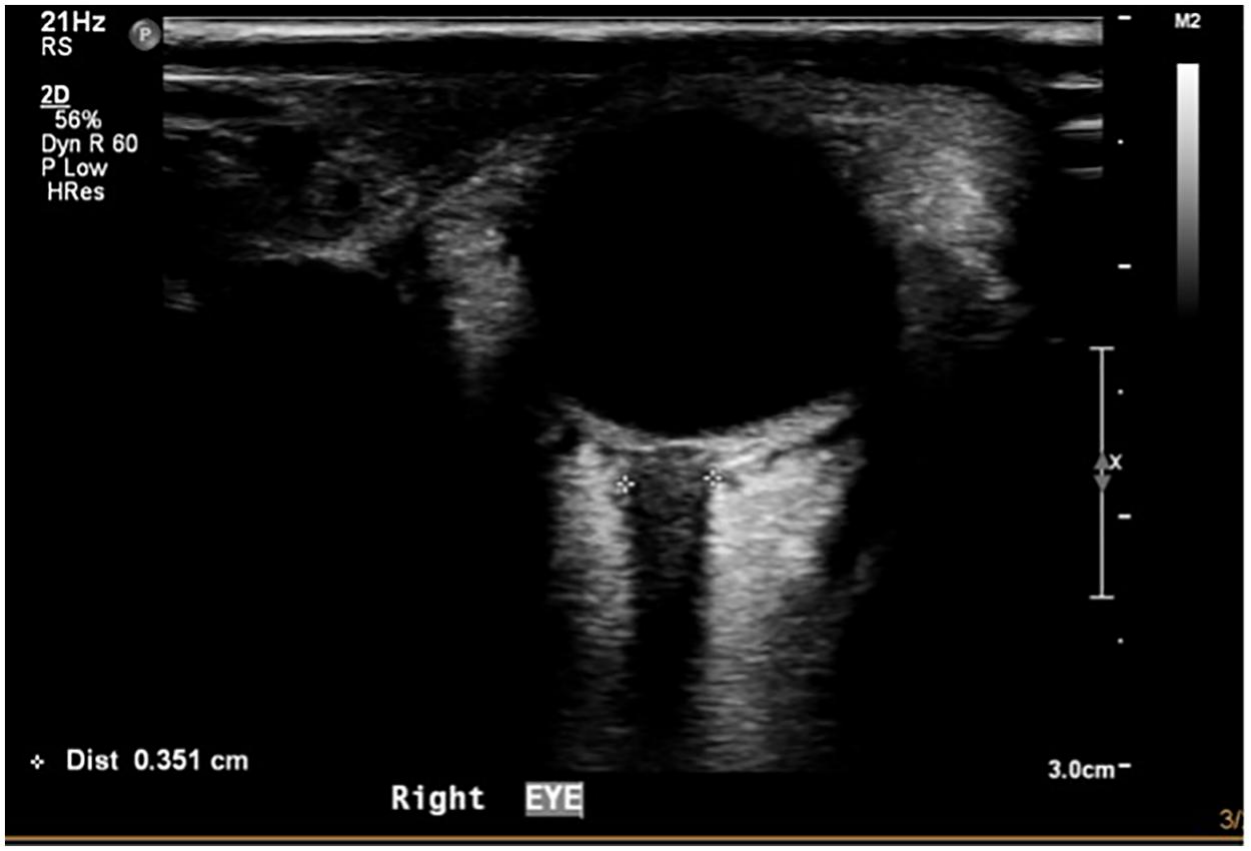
Posterior acoustic shadowing from the LC obscuring ONS margins. This transverse B-mode ultrasound image of the ON demonstrates a common artefact caused by the LC. The resulting posterior acoustic shadow creates a dark vertical band that attenuates the ultrasound beam, obscuring the lateral margins of the ONS. As a result, the external sheath boundaries are not clearly visualised, and accurate ONSD measurement cannot be reliably performed in this image. Recognising this artefact is essential to avoid misinterpretation and potential under- or overestimation of ICP.
